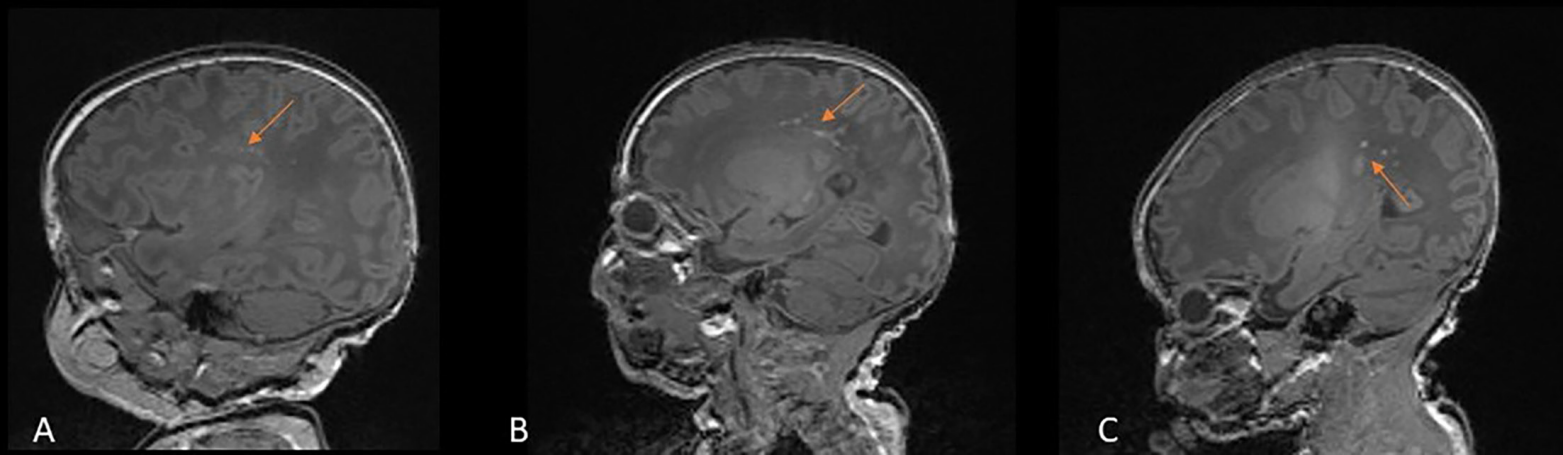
Punctate white matter lesions. T1-weighted images showing punctate white matter lesions in (A) infant aged 32^+0^ weeks, scanned at 44^+2^ weeks, (B) infant aged 31^+3^ weeks, scanned at 39^+0^ weeks, (C) infant aged 39^+1^ weeks, scanned at 40^+5^ weeks.
